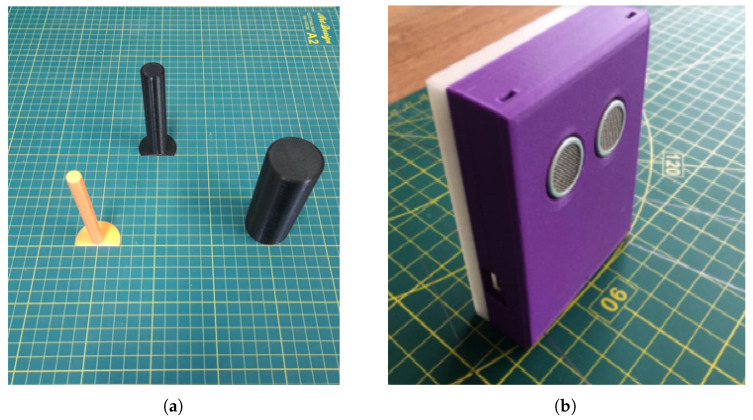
(a) Classified objects; (b) designed ultrasonic sensor.
